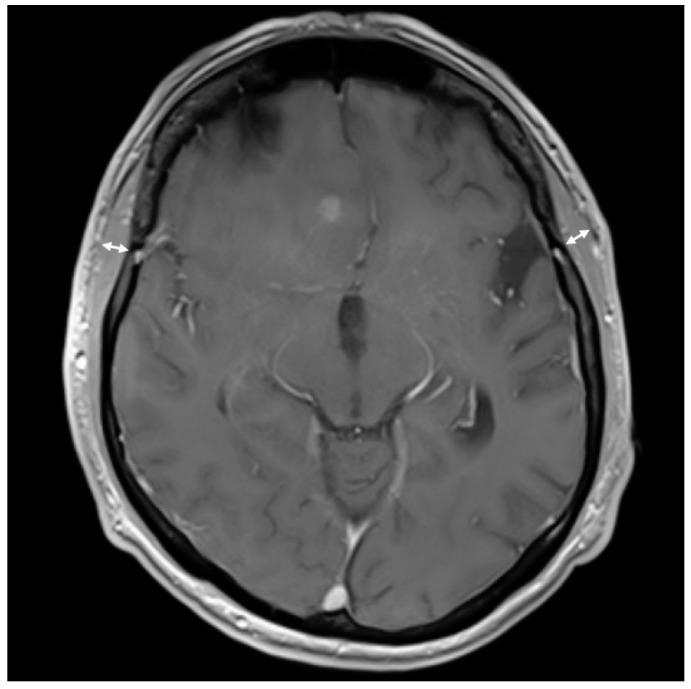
Example of temporal muscle thickness (TMT) measurement in contrast enhanced axial T1 magnetic resonance imaging (MRI) of a 70-year old male patient with an overall survival (OS) of 18 months. Measurement is marked with arrows (right: 7.6 mm, left: 7.8 mm).
